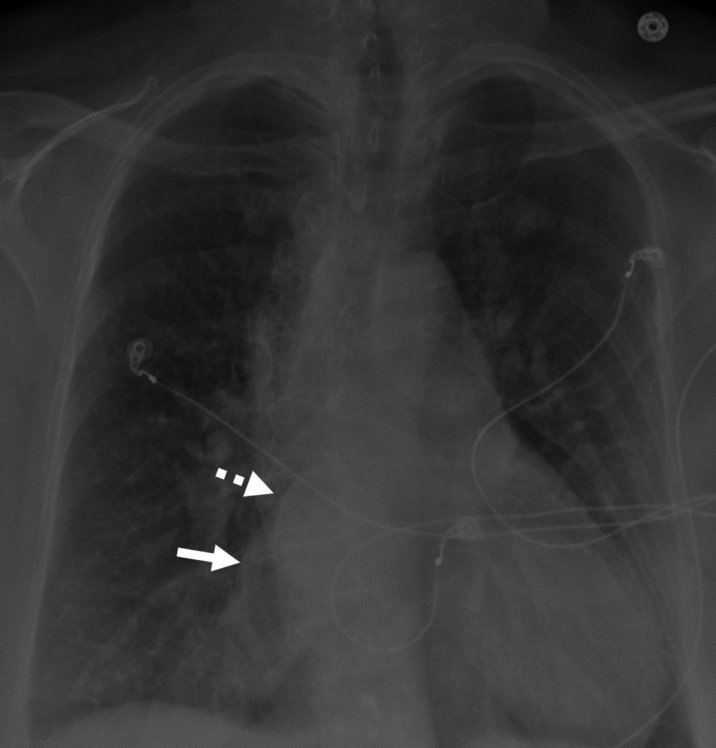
Anteroposterior Chest Radiograph Showing Bilateral Interstitial Pulmonary Congestion and Left (Dashed Arrow) and Right (Solid Arrow) Atrial Enlargement
